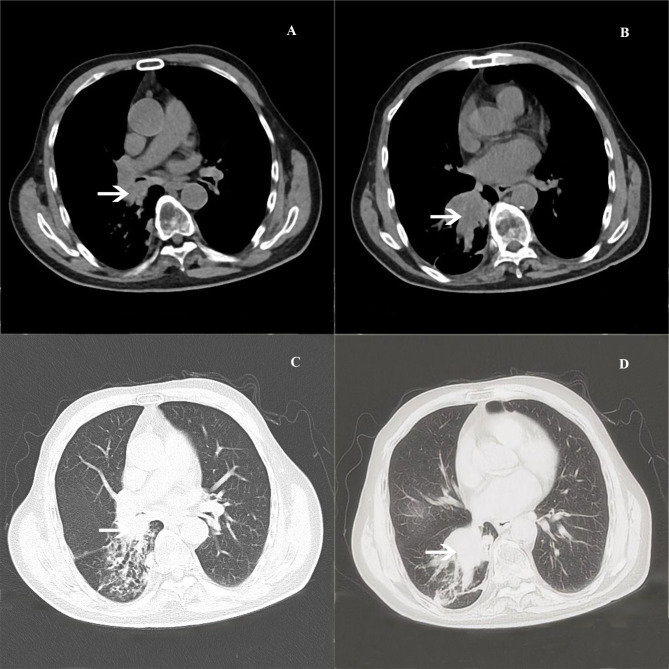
(A–D). Chest CT reveals a soft tissue mass (arrows) distributed along the right main bronchus as well as the right lower lobe bronchi of the lung.
